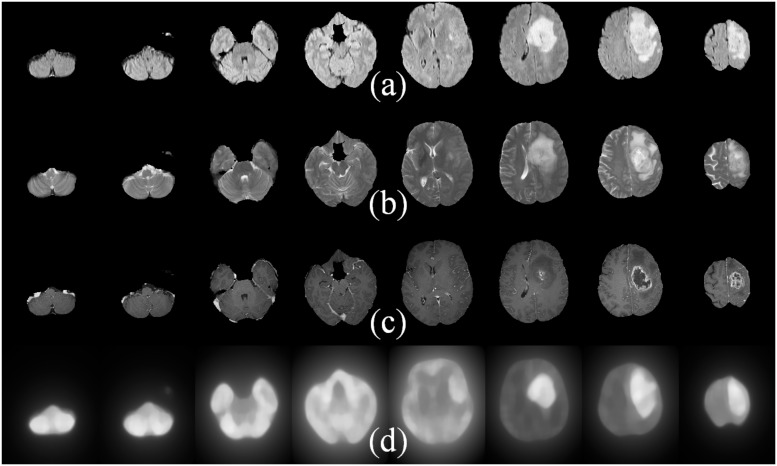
Saliency maps for High-grade glioma samples. MR slice numbers: 13, 19, 35, 46, 67, 81, 100, 121 (left to right) by (a) FLAIR, (b) T2, and (c) T1C sequences. (d) Saliency maps generated in 2D [5].
